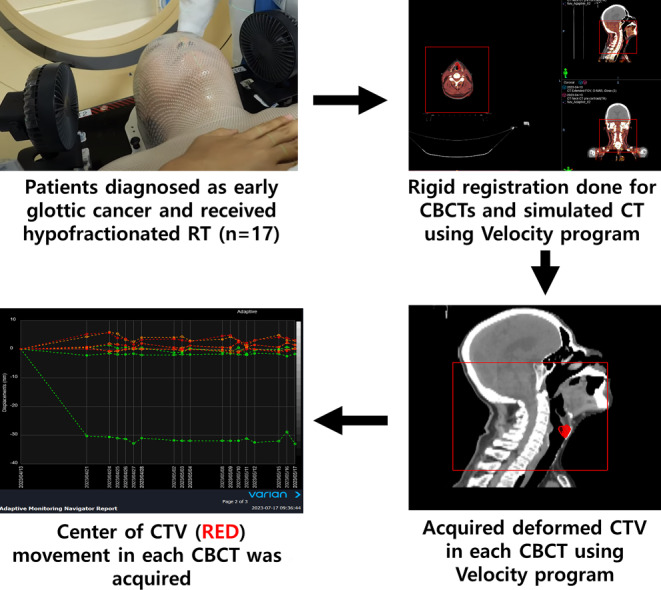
Evaluation of the margin for vocal cord using cone-beam computed tomography. *RT* radiotherapy, *CBCT* cone-beam computed tomography, *CT* computed tomography, *CTV* clinical target volume
